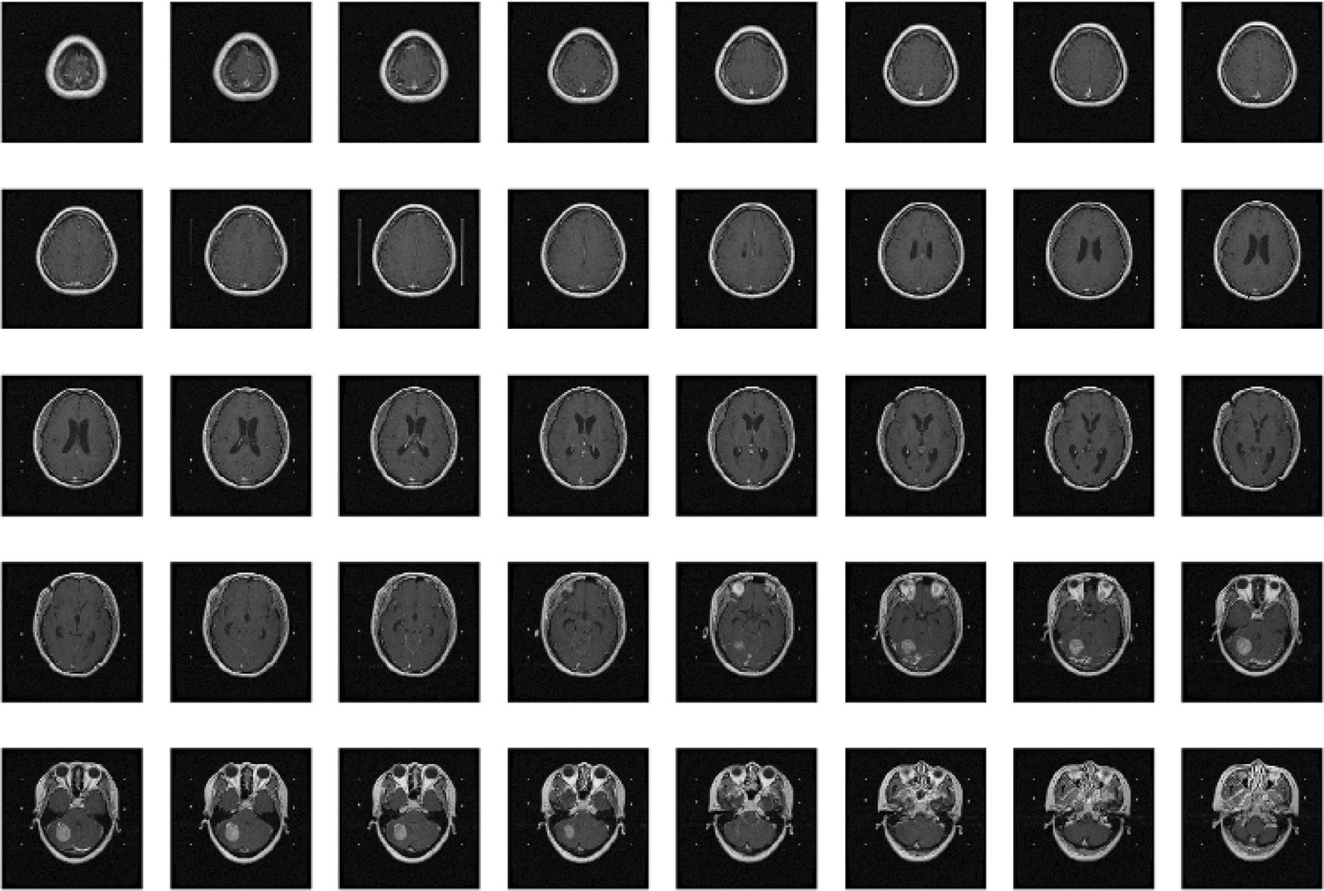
Post-contrast T1-weighted MRI per patient.
